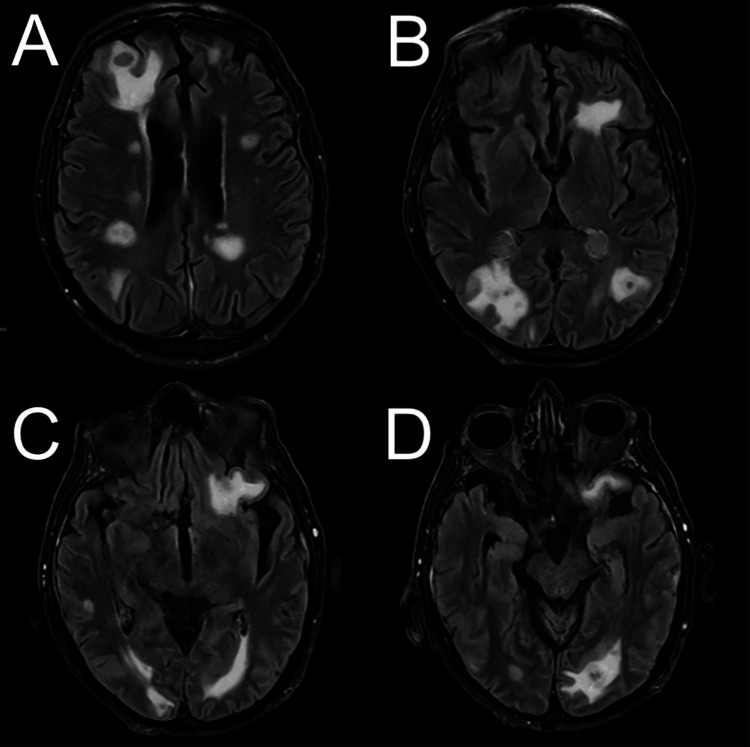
MRI of the brain with and without contrast. T2 flair images demonstrating multiple rim-enhancing lesions through selected sections from most cranial (A) to most caudal (D).
